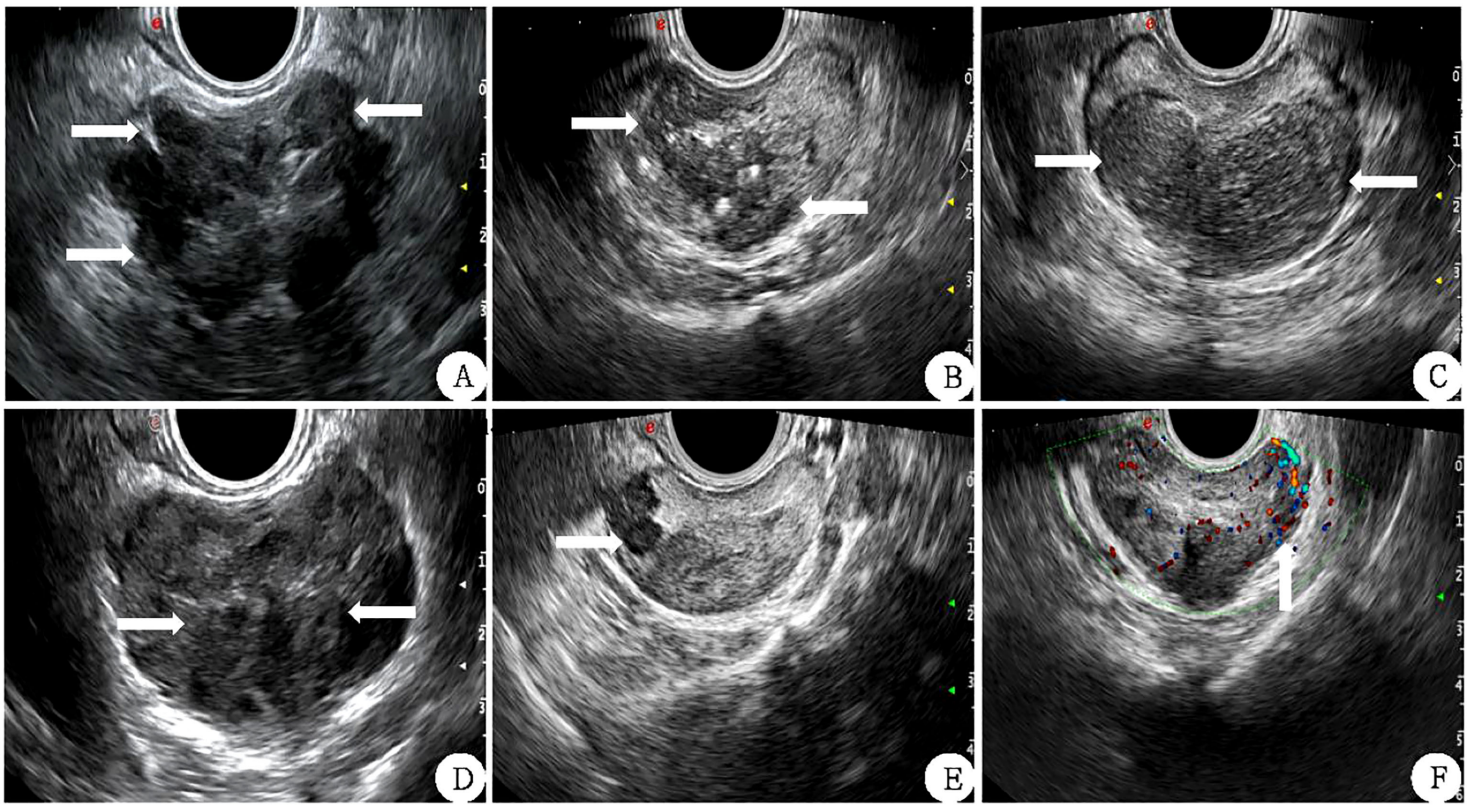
Correlation features of prostate 2D ultrasound image scores (white arrow) (A) The lateral lobe capsule is not smooth and protrudes outwards. (B): the echo of the inner and outer glands is uniform and the boundary is not clear. (C) left and right lobes are not symmetrical. (D) The boundary between the inner and outer glands is unclear. (E) Hypoechoic nodules can be seen in the right external gland. (F) There is abundant blood flow in the left external gland nodule.
